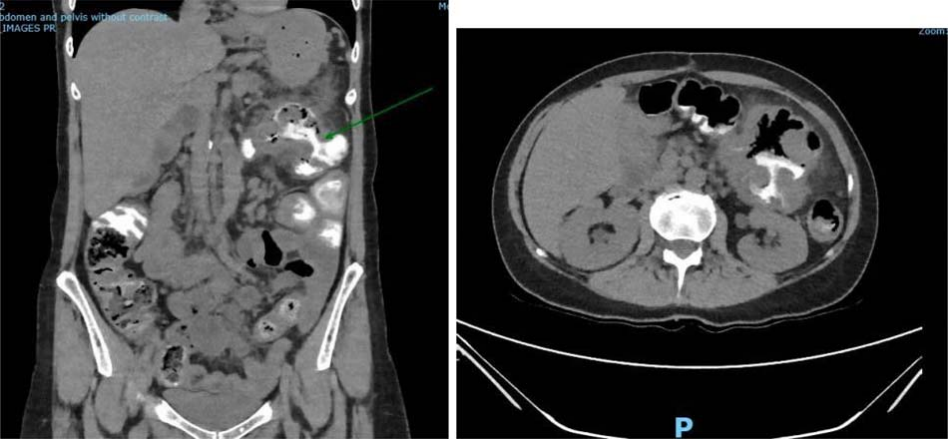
Coronal and axial slices of a CT abdomen/pelvis with oral contrast demonstrating a gastrocolonic fistula.
